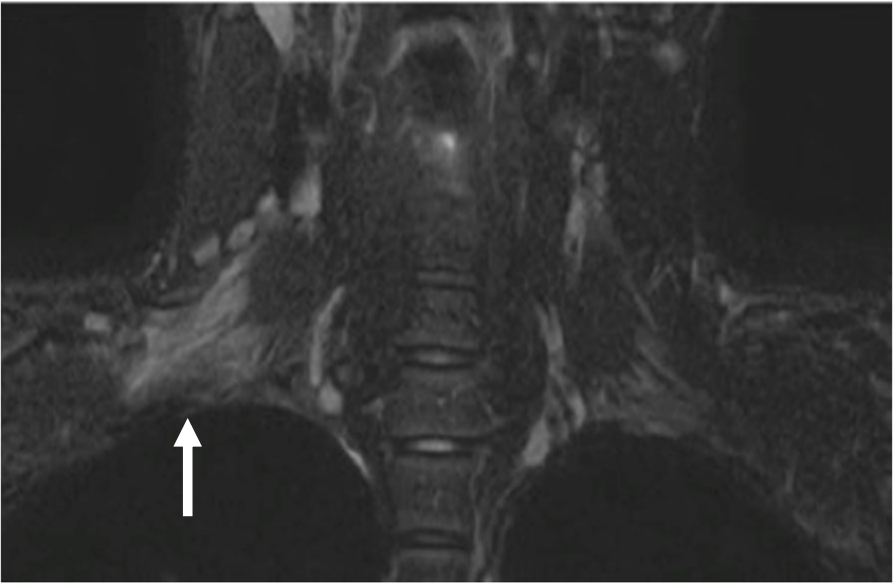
Coronal T2-weighted MRI of the neck at 1 week after surgery showing diffuse nerve enlargement and increased homogeneous signal intensity of the right brachial plexus (arrow). MRI indicates magnetic resonance imaging
